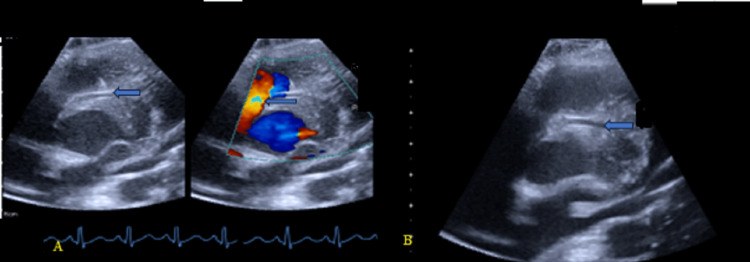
Repeat transthoracic echocardiography demonstrating the anomalous origin of the LMCA from the right aortic sinus. A: 2D and color, B: 2D (blue arrow) LMCA: left main coronary artery
